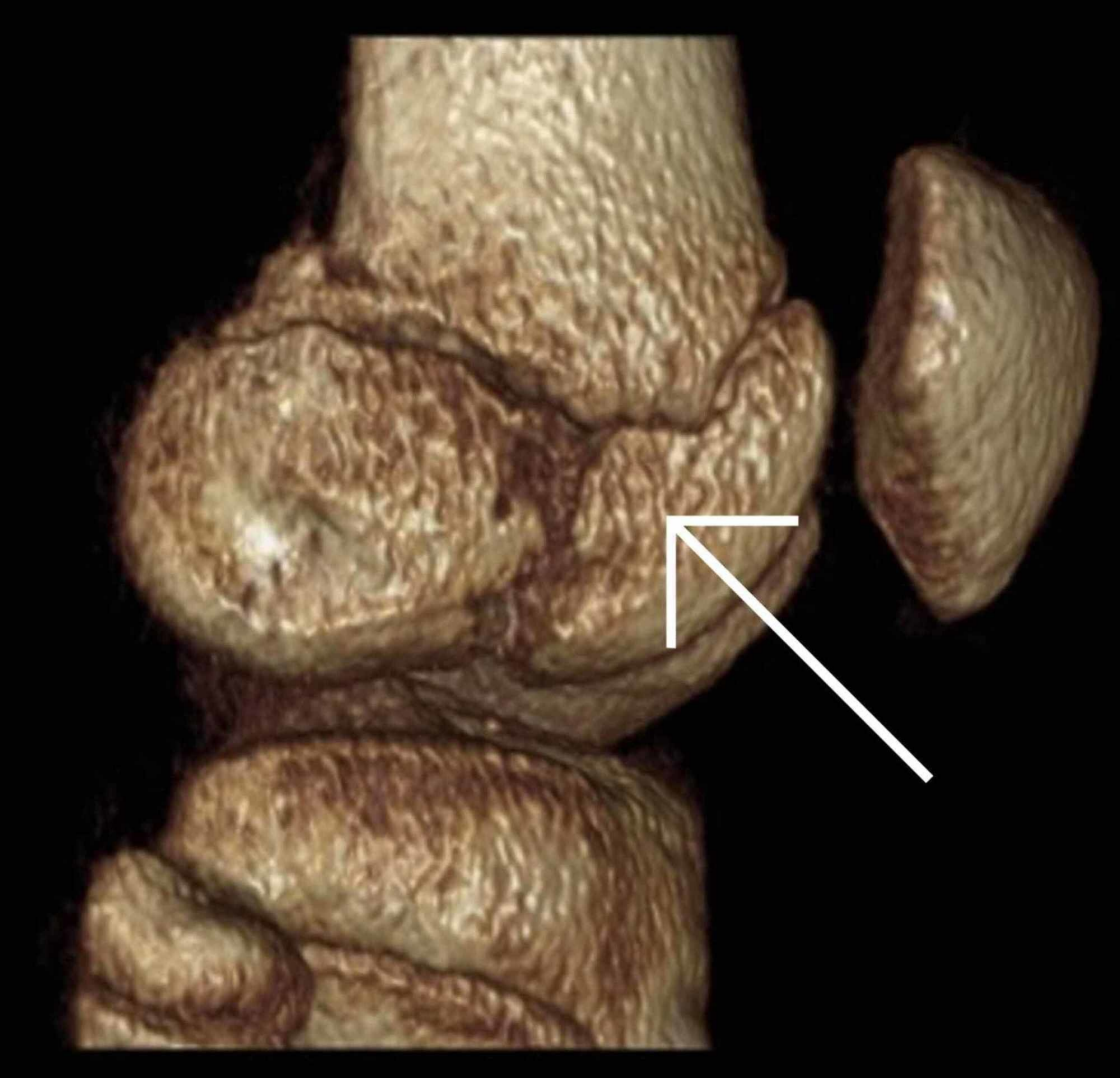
Sagittal view of a computed tomography reconstruction post-injury
